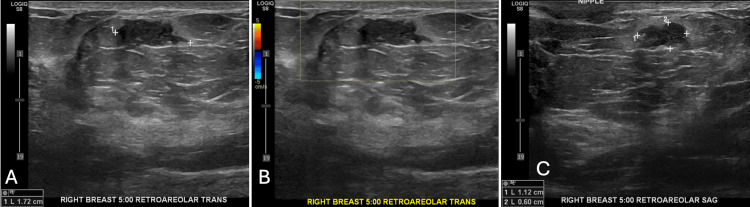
An incidental mass was found on the ultrasound 15 months after the initial screening. Follow-up ultrasound incidentally demonstrated a separate 1.7 cm irregular mass with an angular margin in the retroareolar region. This had intermediate suspicion for malignancy. A. Transverse view. B. The mass had no vascularity on color Doppler ultrasound. C. Sagittal view.
